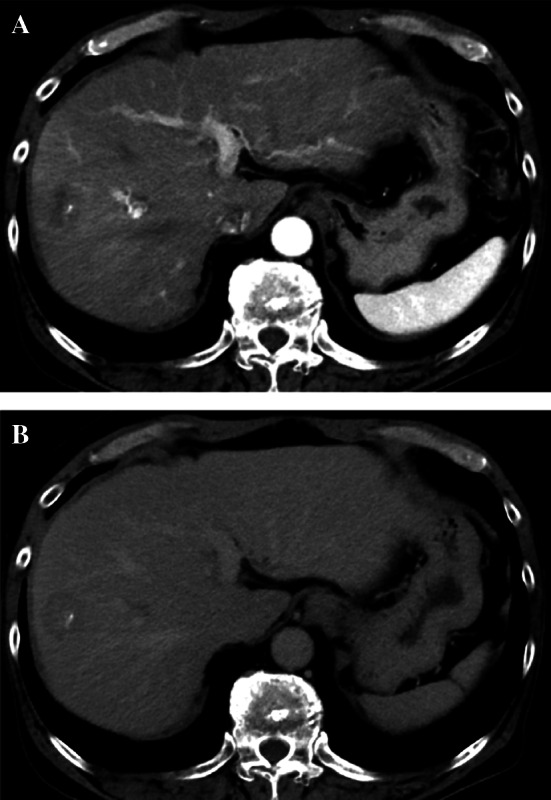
A, B Abdominal contrast-enhanced computed tomography shows a tumor measuring 23 mm in size with ring enhancement in the early phase (arrow). The tumor shows hypointensity in the delayed phase (arrow)
